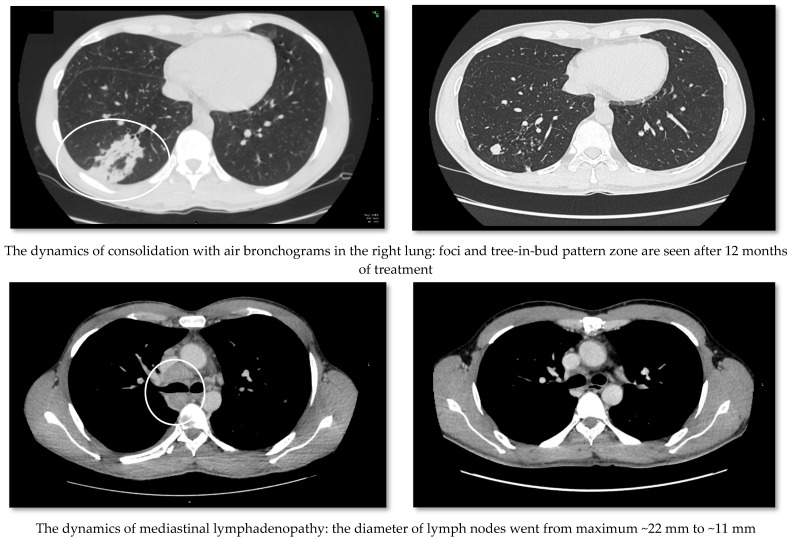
The dynamics of control CT scans before treatment and after 12 months of treatment.
